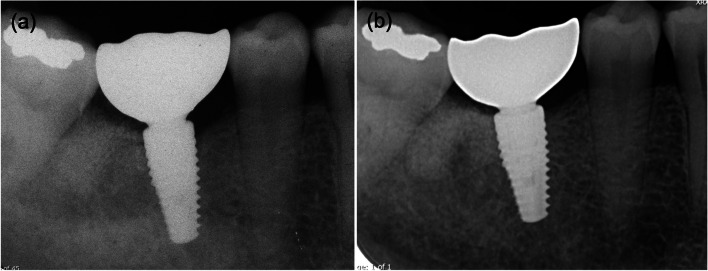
Radiographs of tooth 46: a post-operative radiograph after crown installation; b follow-up at 5 years and 6 months after implant placement
